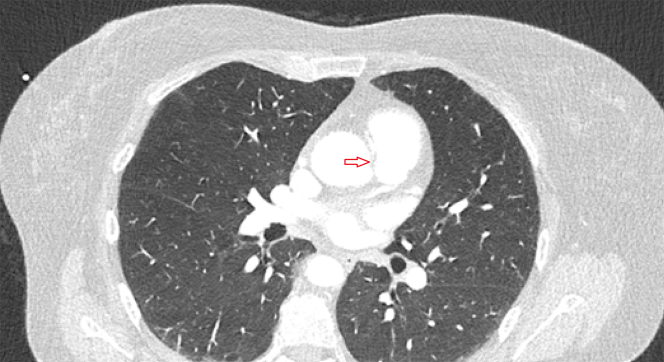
Preprocedural Cardiac Computed Tomography Angiography Axial view of the CCTA demonstrating the AAORCA (red arrow). The vessel originates from the left coronary sinus and follows an interarterial course between the aorta and the pulmonary artery. AAORCA = anomalous aortic origin of the right coronary artery; CCTA = coronary computed tomography angiography.
